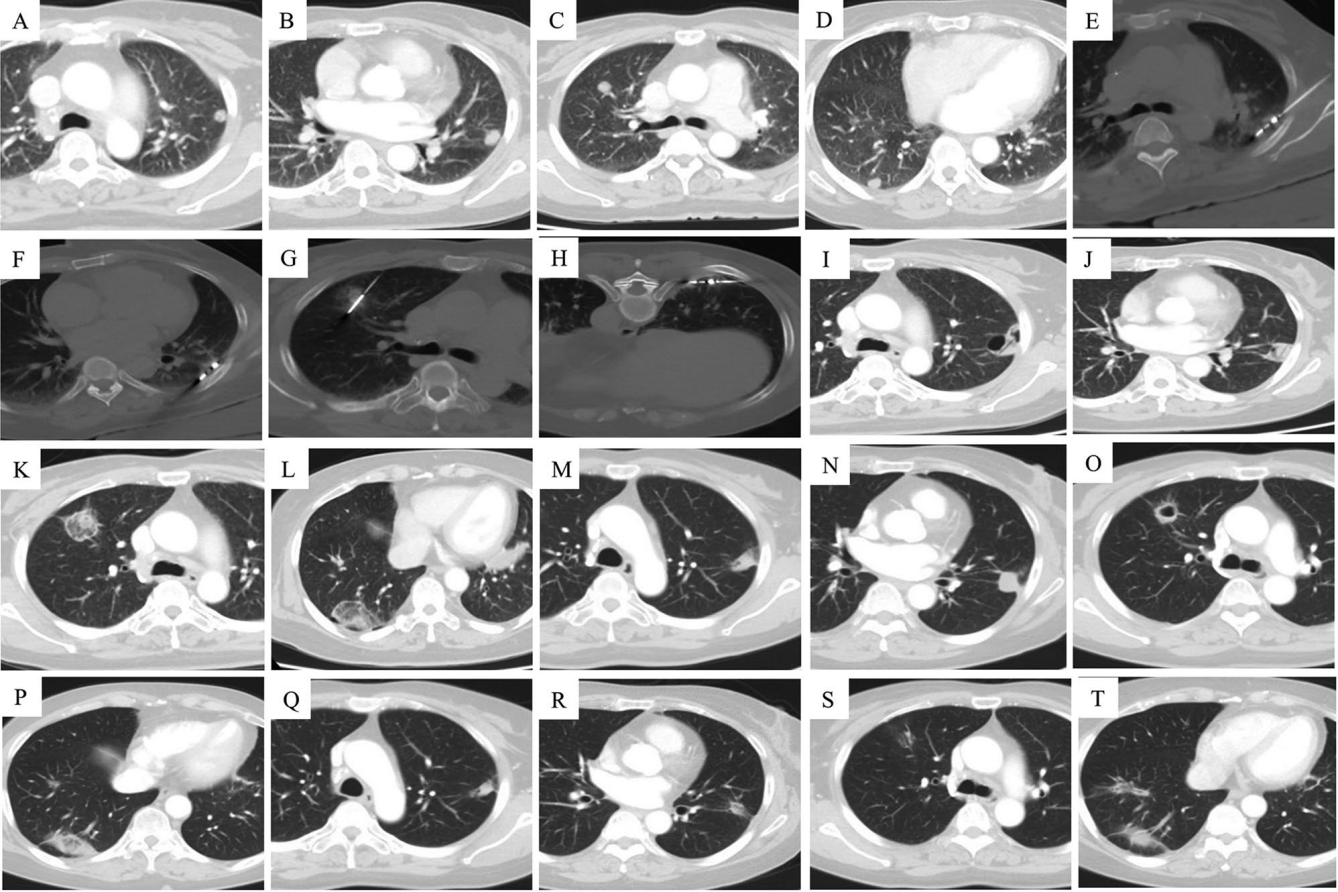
A 63-year-old was found with bilateral lung metastases male 13 months after primary hepatoma carcinoma surgery, and after sorafenib treatment for three months, the lesions’ sizes were enlarged. A Nodules #1 (0.9 × 0.8 cm) and B Nodule #2 (1.4 × 1.1 cm) were observed in the left upper lung lobe. C Nodule #3 (1.4 × 1.1 cm) was observed in the right upper lung lobe. D Nodule #4 (1.3 × 1.1 cm) was observed in the right lower lung lobe. E, F MWA was performed on nodules #1 and #2 under CT guidance. G&H) MWA was performed on nodules #3 and #4 under CT guidance a month after MWA on nodules #1 and #2. I, J 2 months after the MWA session on nodules #1 and #2. K, L 1 month after the MWA session on nodules #3 and #4. M, N 6 months after the MWA session on nodules #1 and #2. O, P 5 months after the MWA session on nodules #3 and #4. Q, R 13 months after the MWA session on nodules #1 and #2, the lesions shrank to fibrous cords with no enhancement. S, T 12 months after the MWA session on nodules #3 and #4, the lesions shrank to fibrous cords with no enhancement. The efficacy was assessed to achieve complete ablation
